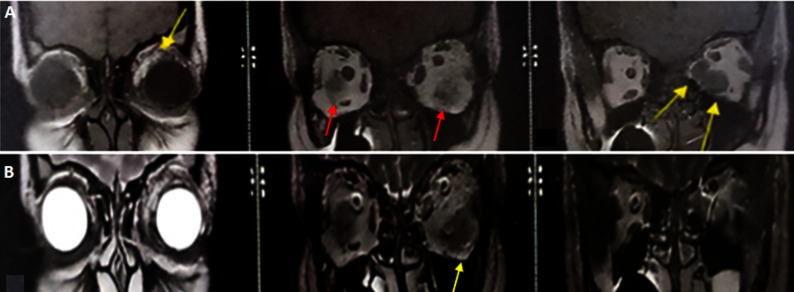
coronal T1-weighted (A) and T2-weighted (B) bilateral ocular proptosis grade I on the right side and grade II on the left side, enlargement and T2-weighted fat-saturated hyperintensity of the left inferior and medial rectus muscles and the left superior oblique muscle, measuring 10 mm, 6.5 mm, and 4 mm, respectively (yellow arrow), bilateral increased orbital fat volume more pronounced on the left (red arrow)
